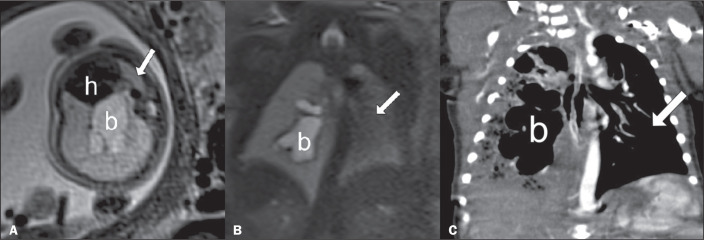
T2-weighted MRI sequences of a fetus with bronchial atresia and a CT scan
